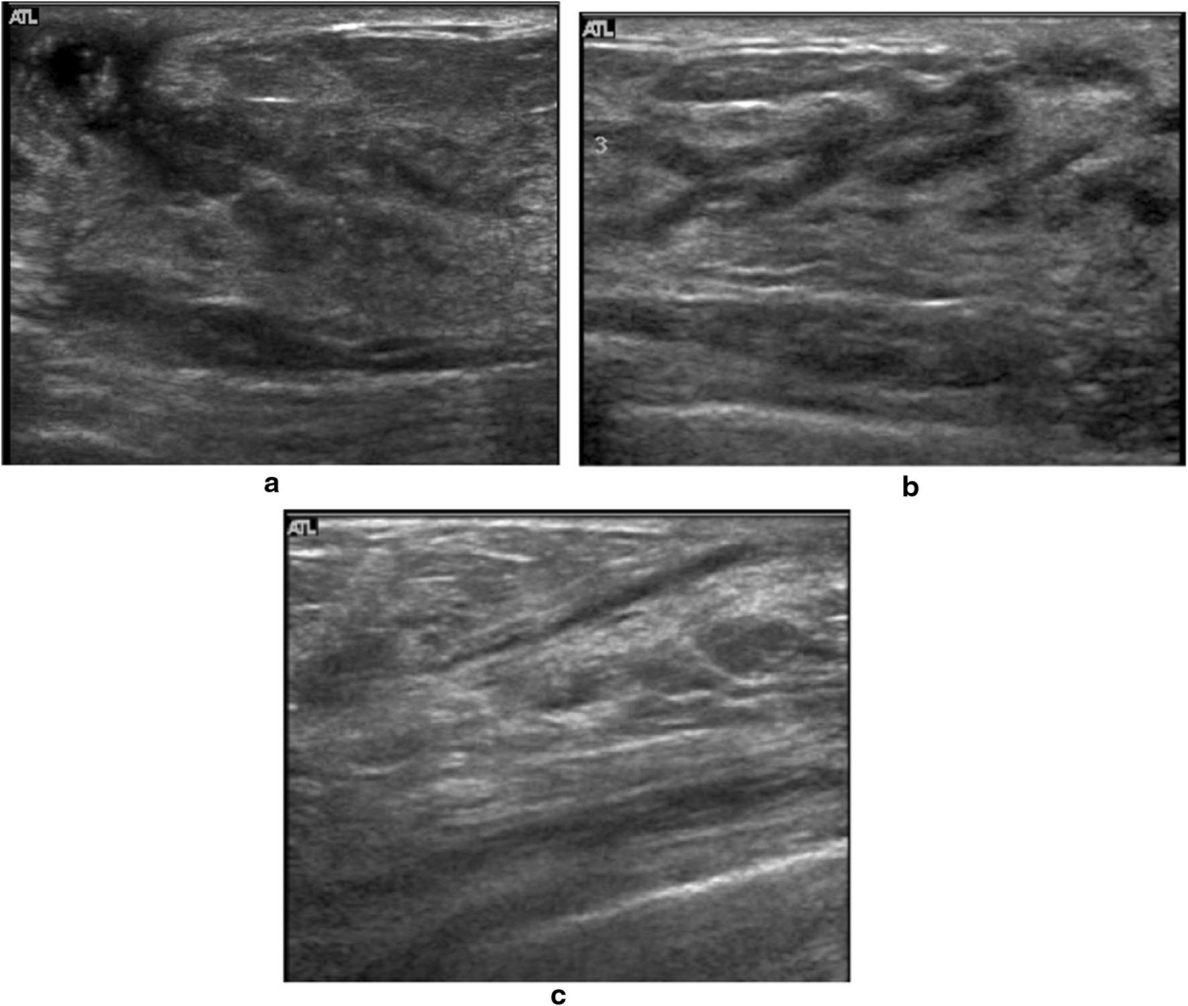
Three cases of ductal carcinoma in situ (DCIS). Ultrasound shows that the beginning is gentle or the solid component in the ducts is continuous
